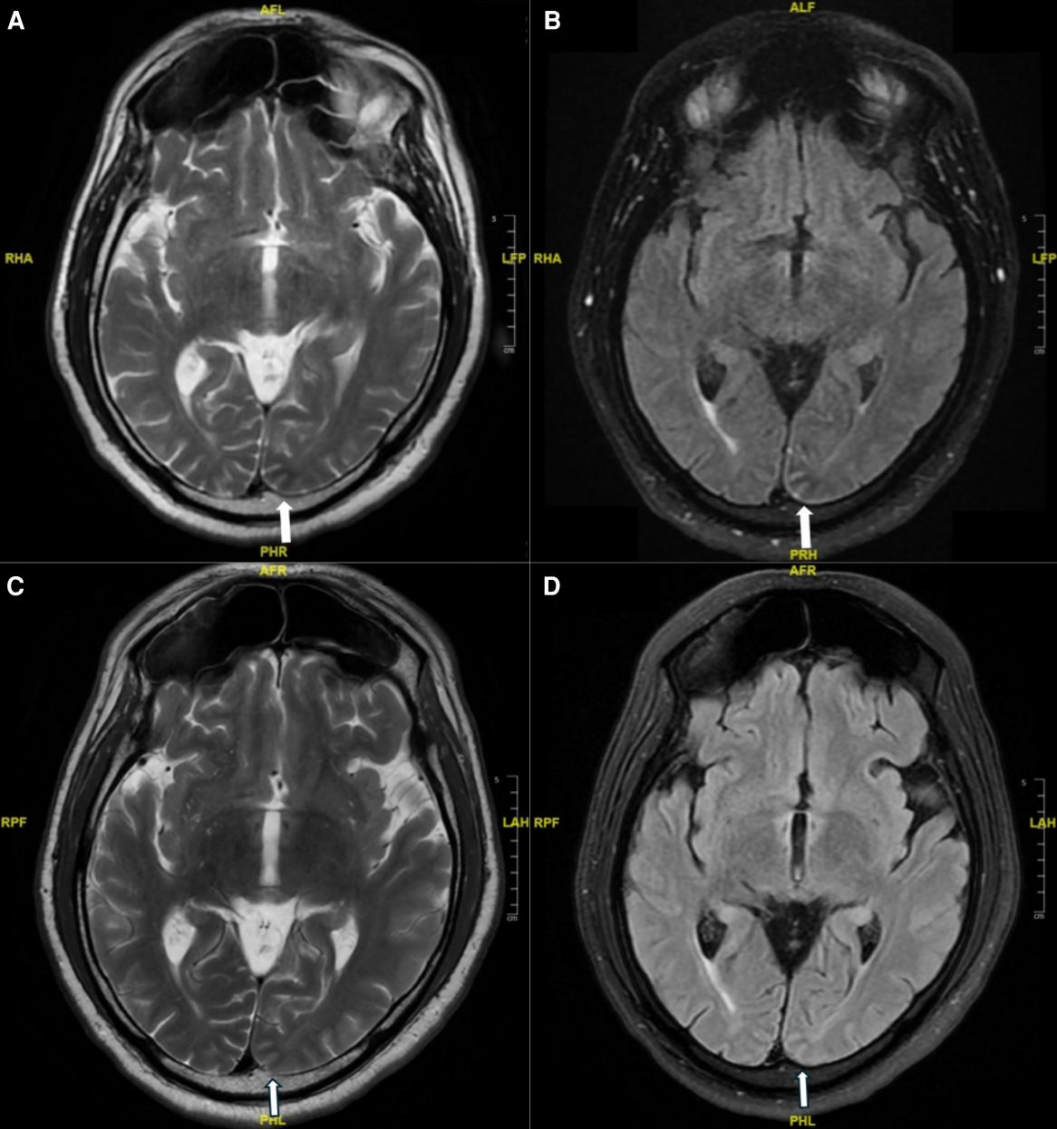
Brain magnetic resonance imaging without contrast demonstrating a subcortical T_2_ hypointensity in the left occipital pole (arrows) at initial presentation (panels A and B) with near-complete resolution on repeat imaging 11 weeks later (panels C and D).
